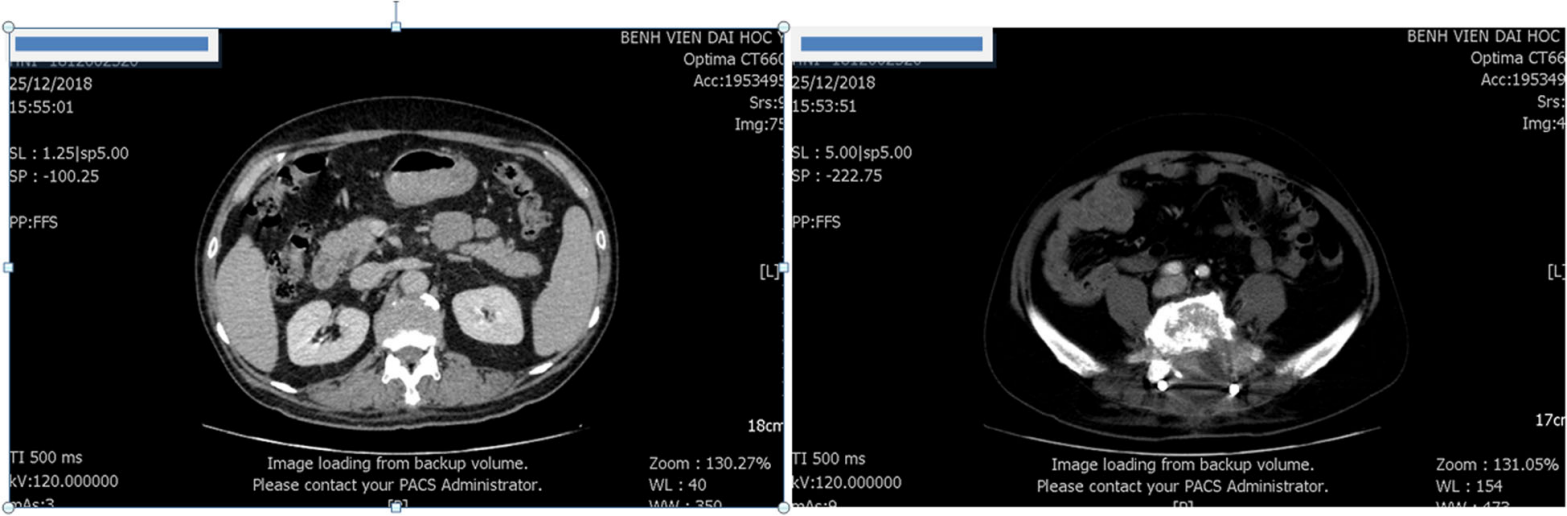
An abdominal computed tomography scan revealed enlarged lytic lesions of S1 with ilium, soft tissue invasion, and compression of the nerve roots from L5 to S2; it did not detect tumor in bilateral kidney (25 December 2018)
